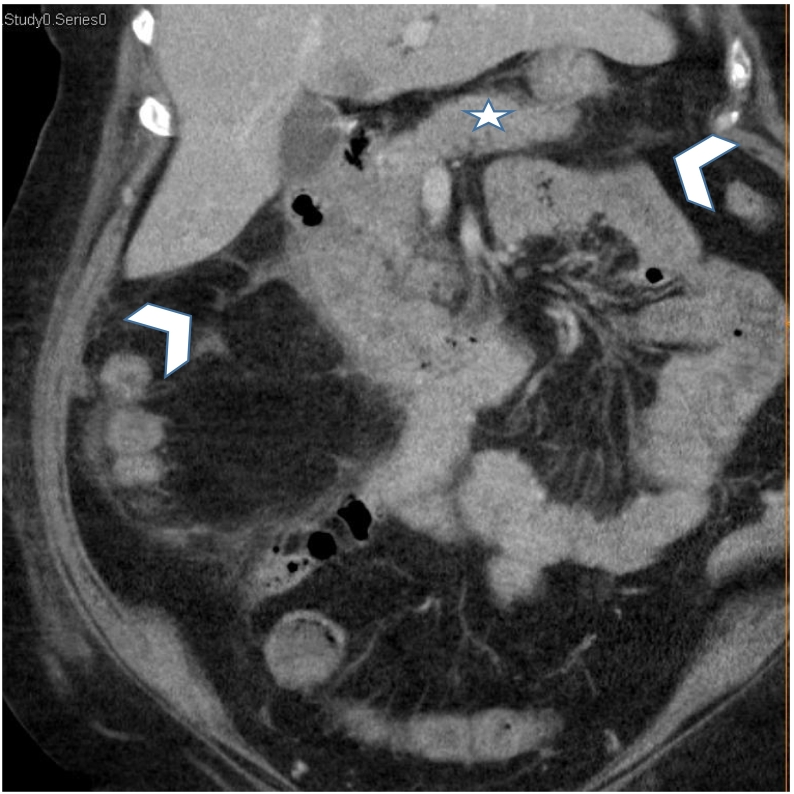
CT coronal reconstructions with contrast injection showing a regression of the regular parietal thickening of the D2, D3 portions of the duodenum and the densification of the mesenteric fat around (arrowheads), the pancreas returns to its normal size (star).
